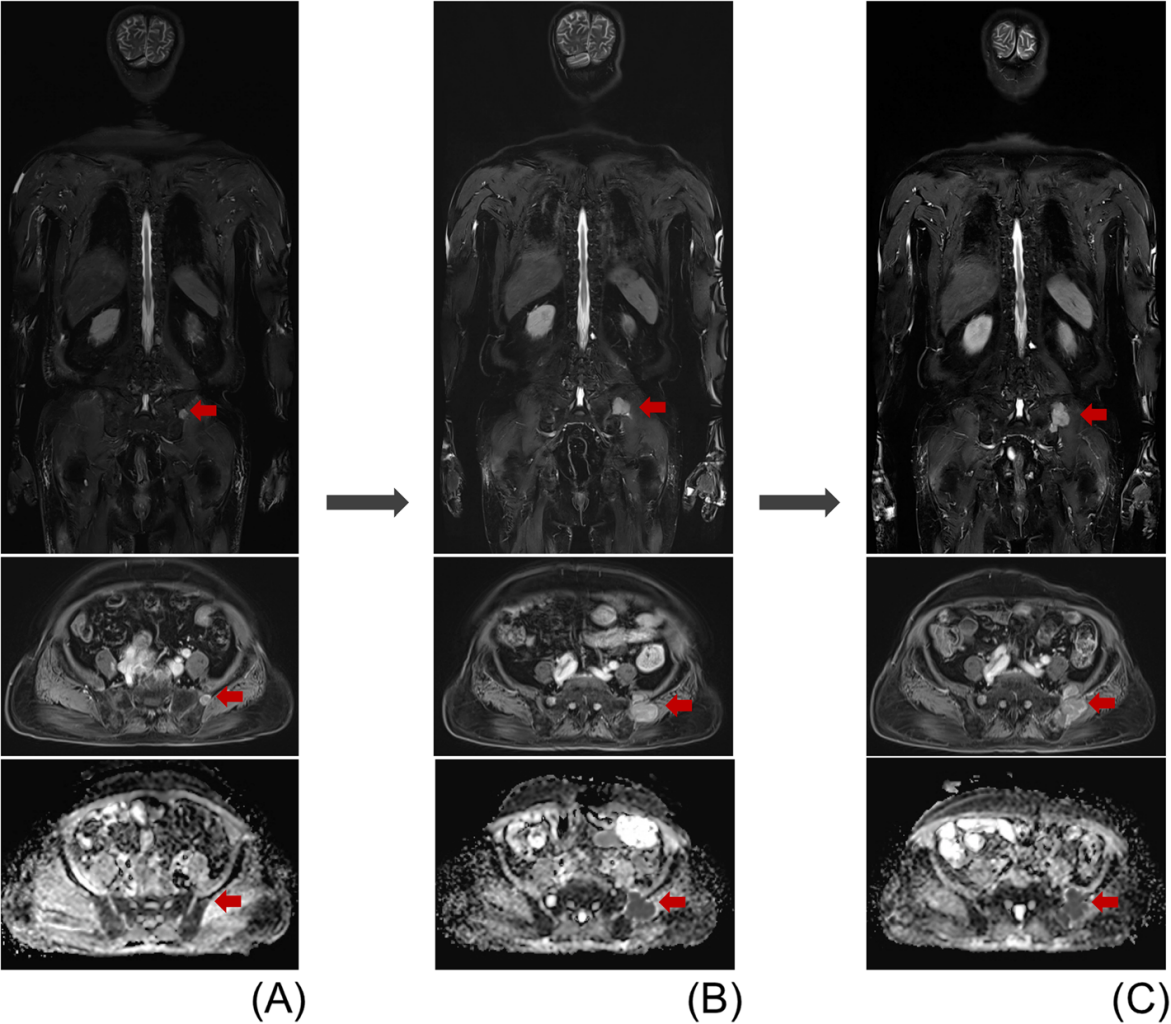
A 70-year-old male with multiple myeloma demonstrating a discrepancy between imaging response and clinical response. MRI taken before chemotherapy (a) shows a nodular bone marrow lesion in the left ilium with T2 hyperintensity (upper right) and contrast enhancement (middle right). It shows diffusion restriction on ADC map (mean ADC value: 0.93 × 10^− 3^ mm^2^/s) (lower right). Follow-up MRI at 3 months (b) and 8 months (c) show gradual increase in the size of the bone marrow lesion with extraosseous soft tissue extension involving the left iliacus and gluteus medius. Aggravation of diffusion restriction was noted on follow-up ADC maps (mean ADC value: 0.70 ~ 0.78 × 10^− 3^ mm^2^/s). Clinical markers improved over the same period (serum M-protein: 2.1 ➔ 0 g/dl); however, despite the improvement in the laboratory markers, the treatment regimen was changed according to the disease progression on the imaging response
